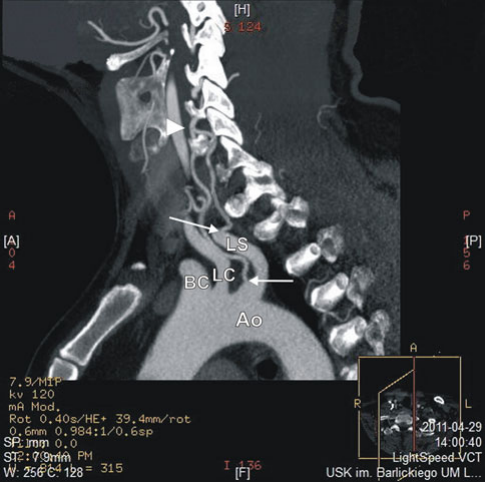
Helical CT angiography, MPR reconstruction in sagittal plane of the neck and superior part of the thorax: *arrows* origins of the duplicated left vertebral arteries, *arrowhead* level of fusion of duplicated left vertebral arteries, *Ao* aortic arch, *BC* brachiocephalic trunk, *LC* left common carotid artery, *LS* left subclavian artery
